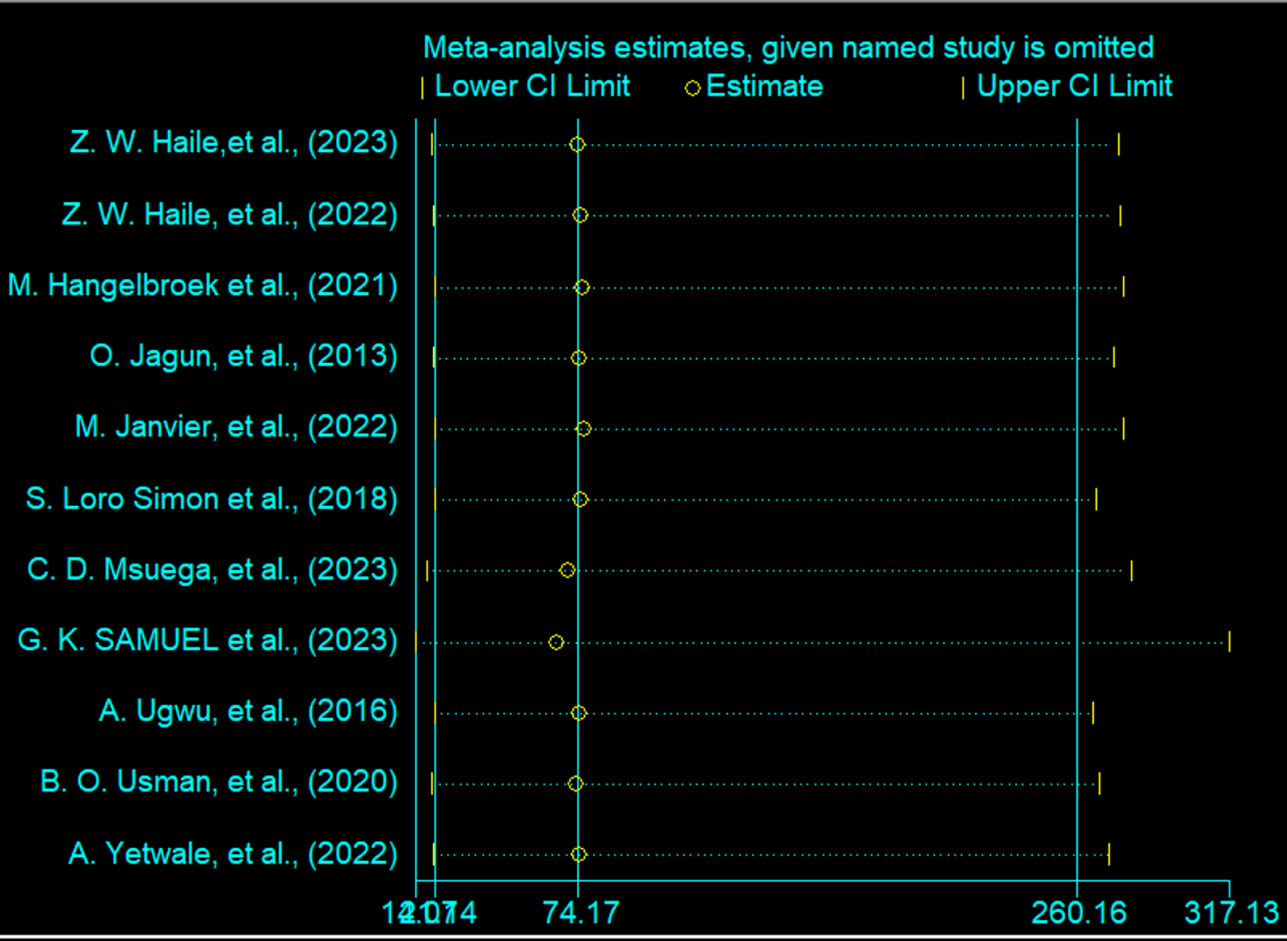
This forest plot presents a sensitivity analysis of the pooled proportion of utilization of obstetric ultrasound among women in Africa
